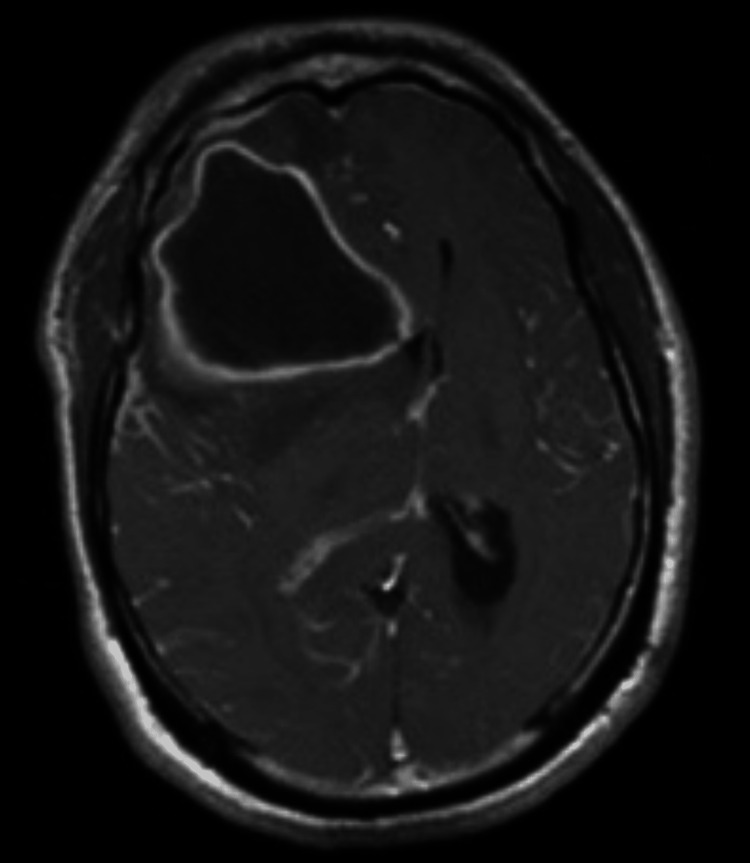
T1-weighted post-gadolinium demonstrating right frontal lobe mass, hypointense center with a thin enhancing rim.
